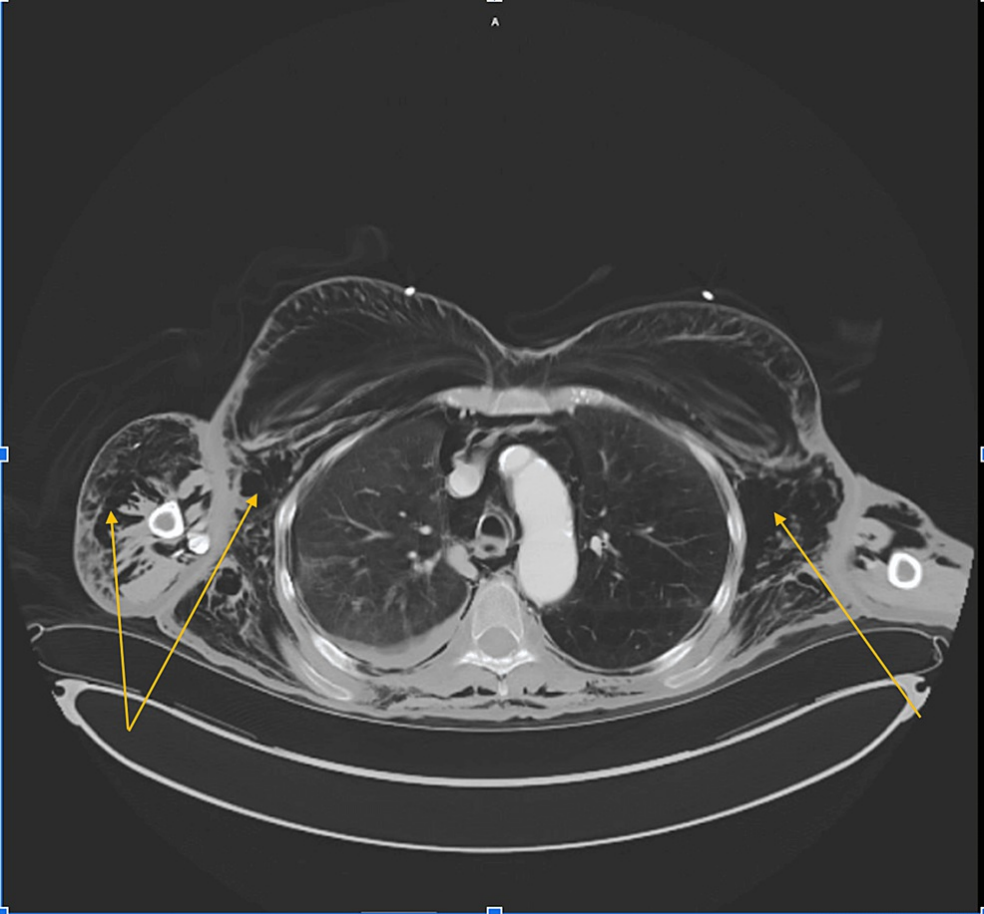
Computed tomography (CT) of the chest/abdomen/pelvis with intravenous contrast - axial view CT abdomen/pelvis in the lung window. The image displays extensive soft-tissue subcutaneous emphysema (yellow arrows).
